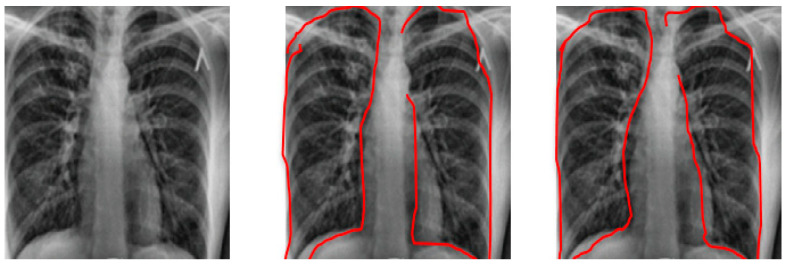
X-ray radiography, with segmented Pulmonary original BoxENet and Segmented Pulmonary Improved BoxENet.
